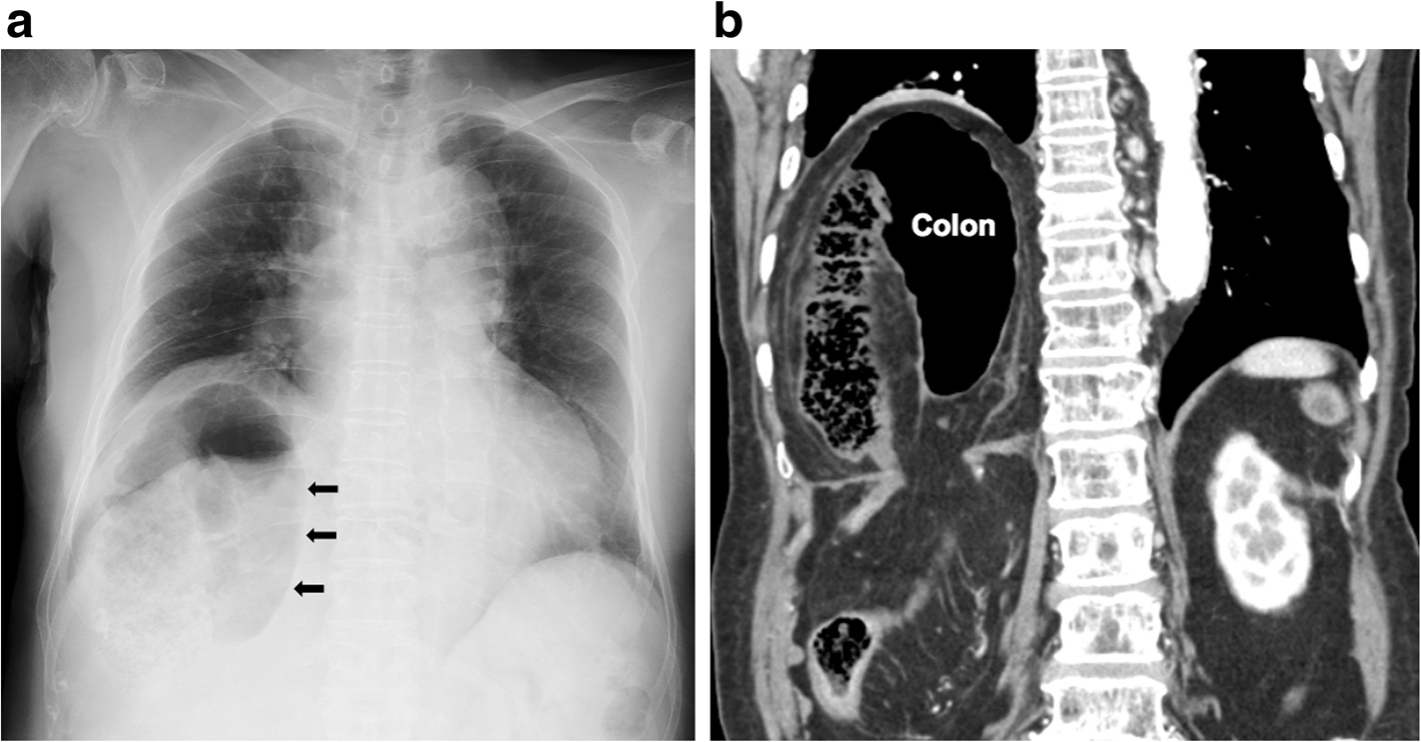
Chest X-ray showing the dilated colon above the right diaphragm (a). CT scan showing strangulation of the colon with some ascites fluid in a sac (b)
